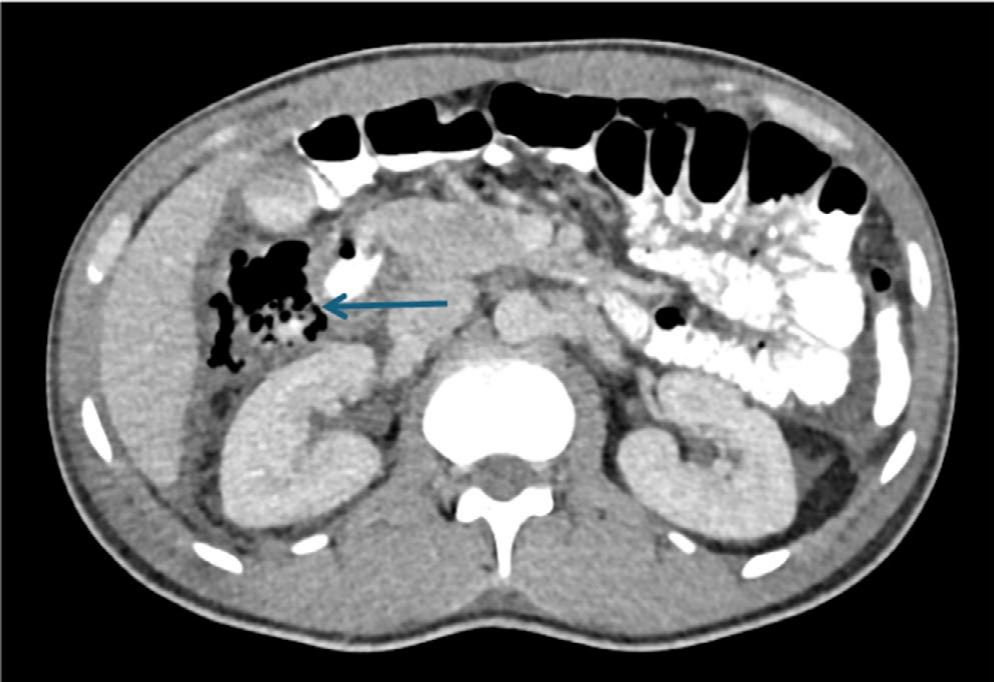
Evidence of pneumoretroperitoneum below the liver and around the duodenum in computed tomography imaging (arrow).
